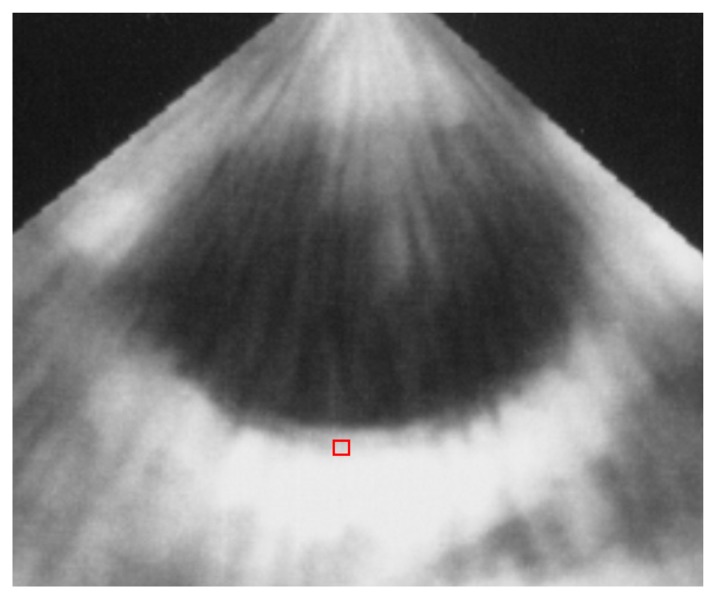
Acquisition of IB value of carotid artery using transthoracic echocardiography. Red square: region-of-interest (11 × 11 pixels).
